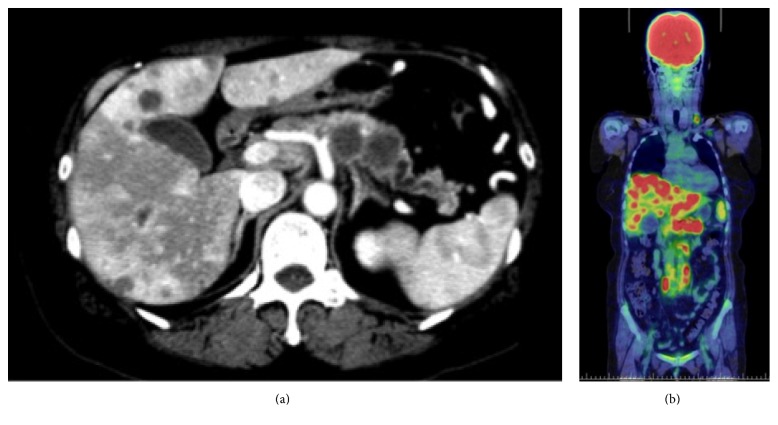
Computed tomography findings. (a) Contrast-enhanced computed tomography showed a hypovascular tumor in the pancreatic body and multiple liver masses with marginal enhancement. (b) Positron emission tomography-computed tomography showed accumulation of ^18^F-fluorodeoxyglucose in the pancreatic body mass [maximum standardized uptake value (SUV max), 7.6], liver masses (SUV max, 9.4), swollen lymph nodes (SUV max, 8.4), and lung masses (SUV max, 1.0).
